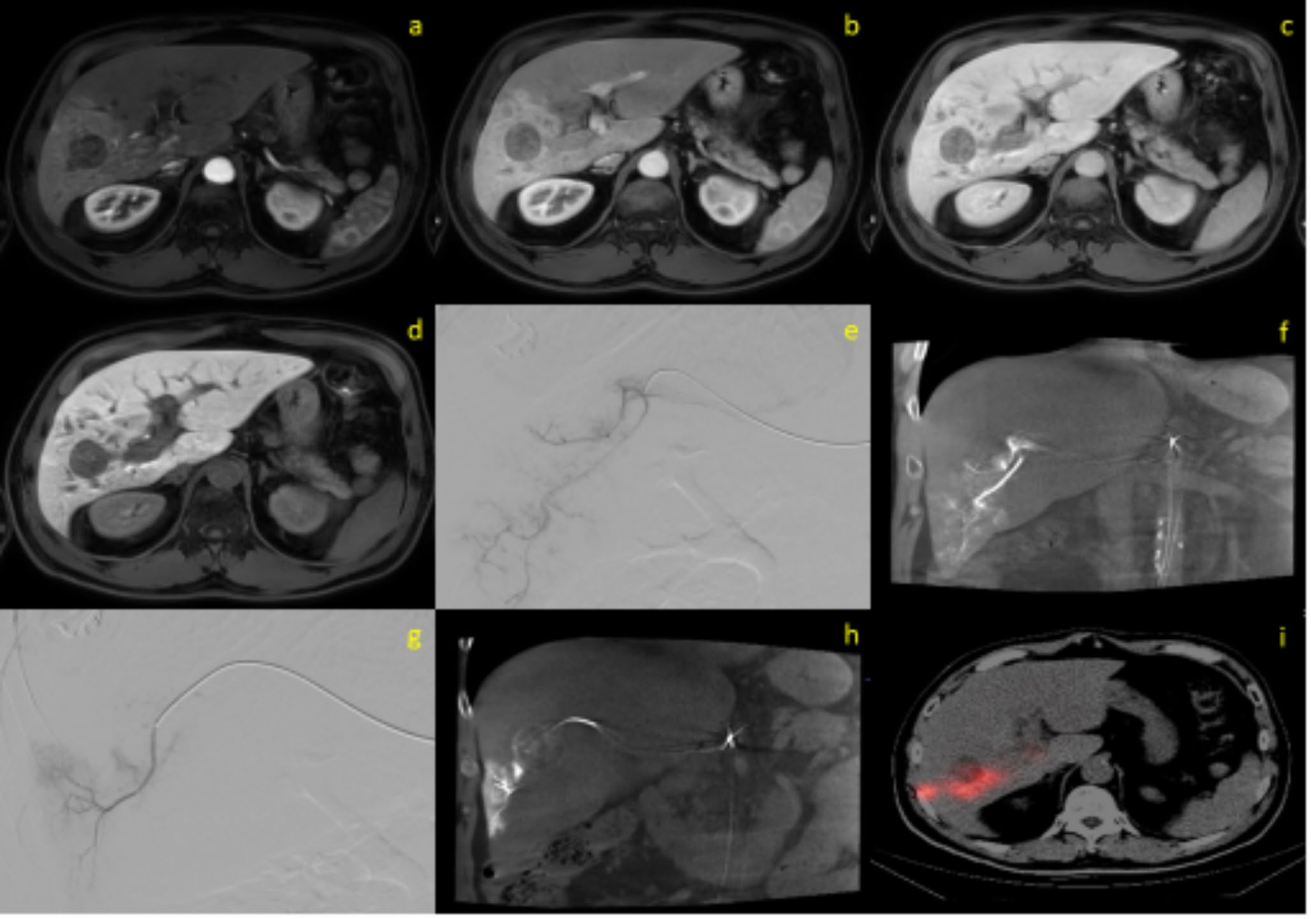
A 53-year-old patient with an 34 mm HCC lesion in segments 5–6 was deemed unsuitable for treatment following split-dose MAA injection through two separate segmental arteries. (a-d) Arterial phase (a), Portal venous phase (b), Venous phase (c) and Hepatobiliary phase (d) contrast-enhanced T1-weighted MRI image of the lesion in segment 5–6; (e, f) Angiographic (e) and cone beam CT (f) images of the segmental artery where the first MAA was administered; (g, h) The segmental artery in which the second MAA was administered, depicted in both angiographic (g) and cone beam CT (h) images; (i) SPECT/CT image demonstrating MAA uptake predominantly in the posterior part of the lesion and within the normal parenchyma, without complete coverage of the entire mass. (CT: Computed tomography; HCC: Hepatocellular carcinoma; MAA: Tc-99 m-macroaggregated albumin; MRI: Magnetic resonance imaging; SPECT: Single-photon emission computed tomography)
